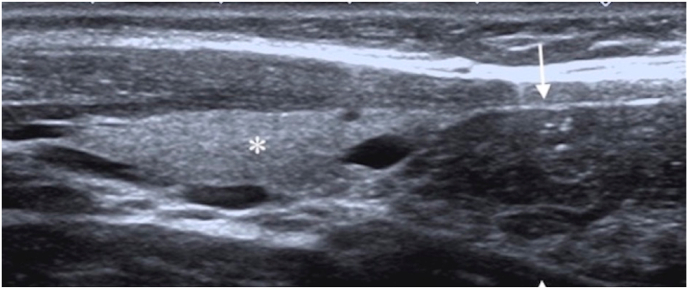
a. The ultrasonography image acquired on the transverse plane reveals cervical extension of the thymus (arrows) located anterior to the cervical trachea (asterisk) in a one-year-old boy. The thymic length was measured on the same plane in mm. Note the typical ‘starry sky’ appearance of normal thymus tissue. Fig. 1b. On the longitudinal plane, the cervically extended thymus tissue (arrows) is just below the thyroid lobe (asterisk).
